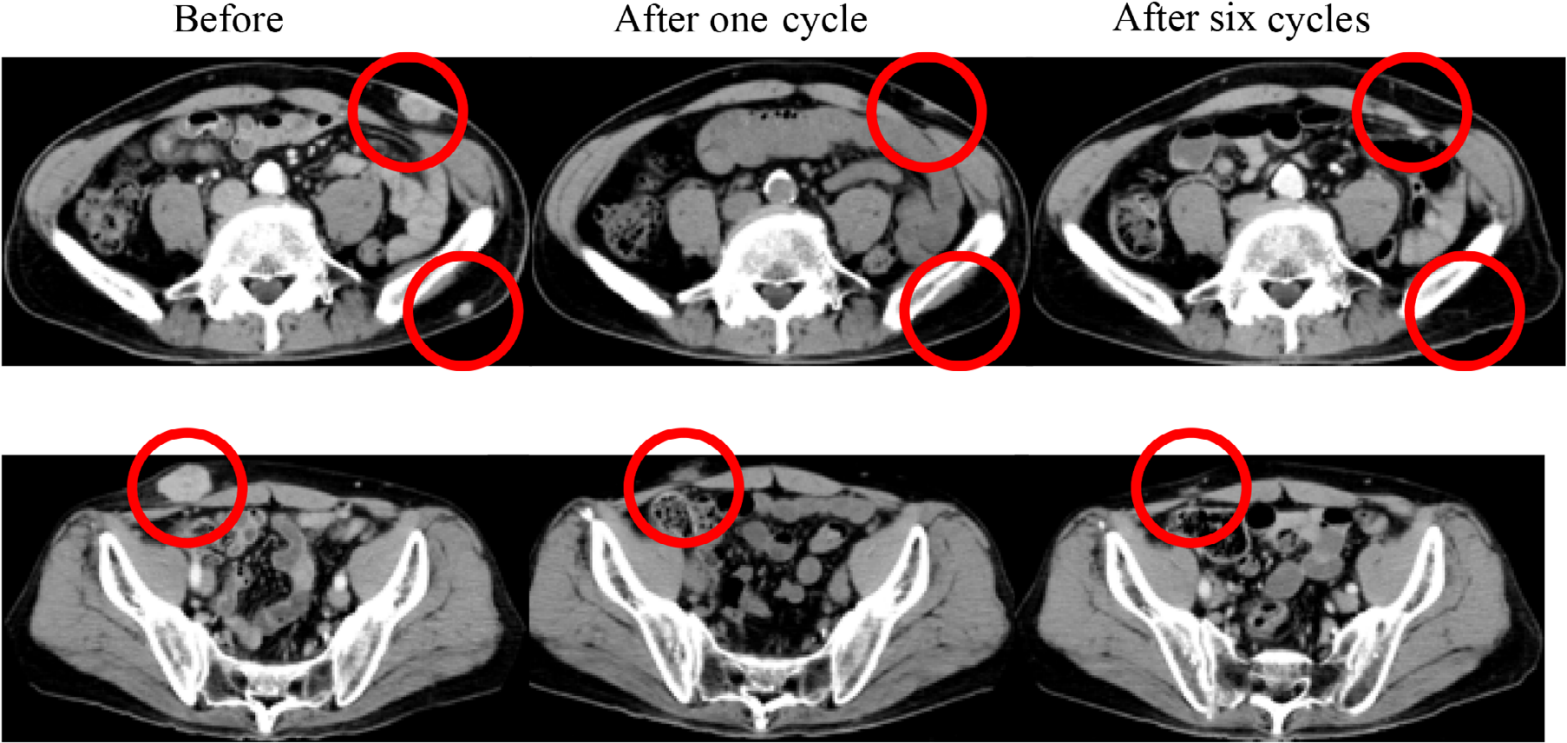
CT after pembrolizumab monotherapy. The tumor had completely disappeared after six cycles
